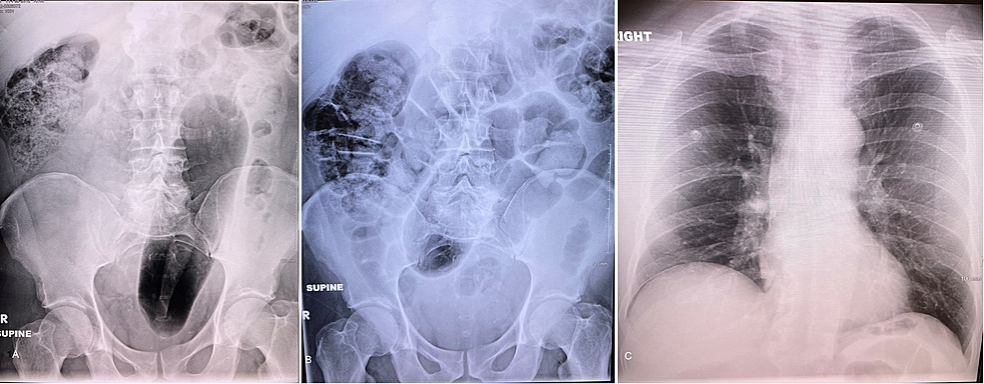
Abdominal and chest X-Ray: A. Abdominal X-ray image with a large area of hyperlucency suspicious for a foreign body in the colon. B. Repeated abdominal X-ray image shortly after the removal of the RFB. C. Chest X-ray image after removing RFB and before discharge. RFB, rectal foreign body.
